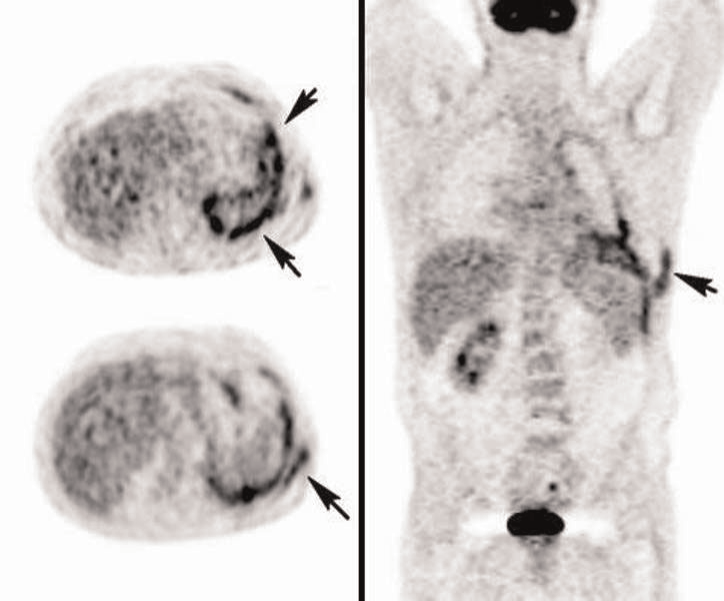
FDG-PET showing striking hypermetobolism in the pleural cavity and chest wall lesions.
